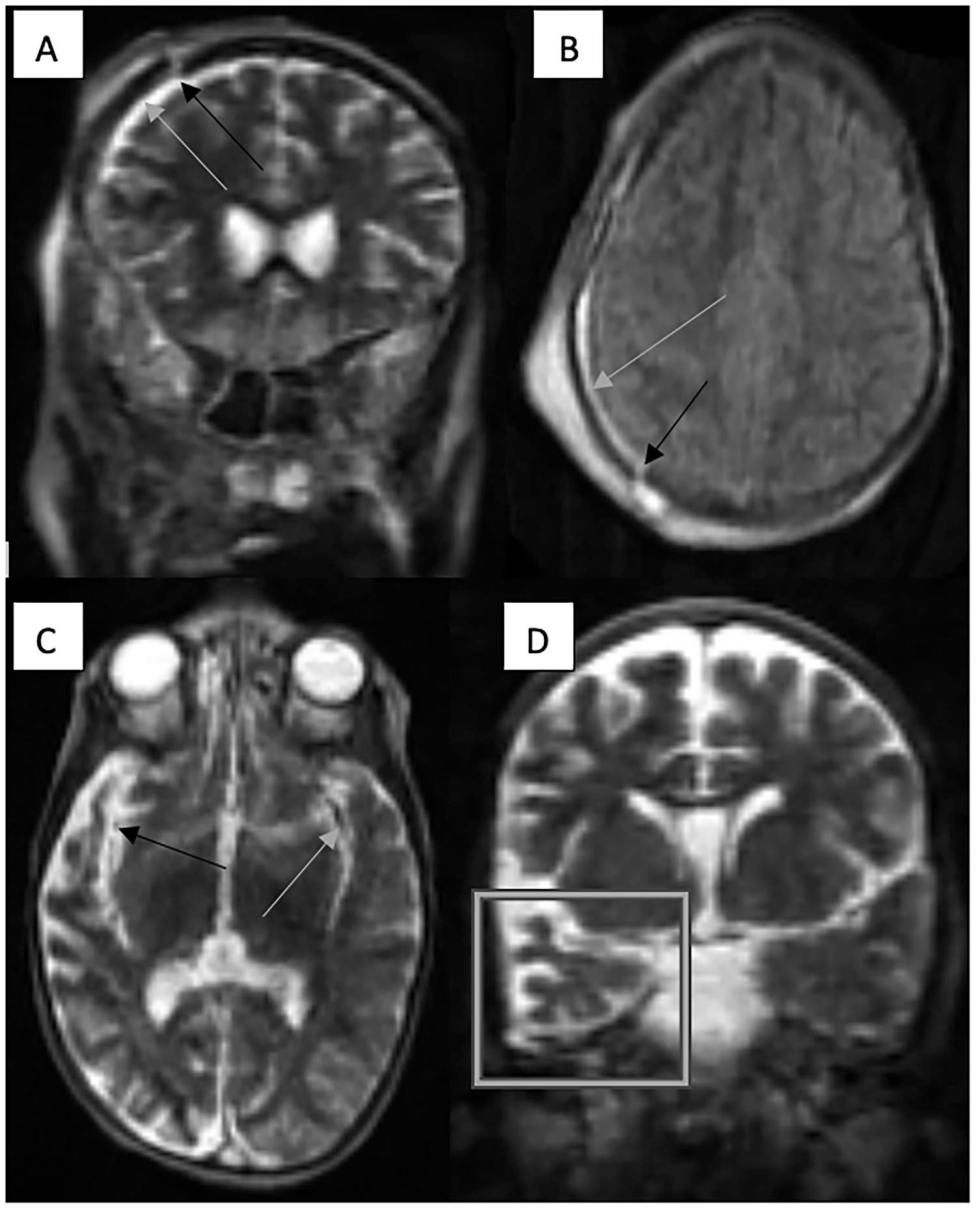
Post traumatic findings in Panels A and B and post ischaemic event sequelae in Panels C and D as imaged on the LF-MRI. Mildly depressed right frontal skull fracture (indicated by the black arrows in Panels A and B) with an overlying scalp oedema/haematoma and underlying widening of the underlying T2W and FLAIR hyperintense subdural fluid space (suspected to be a haematoma) (indicated by the grey arrows in Panels A and B) on the coronal T2W and axial FLAIR sequences. Global atrophy of the grey and white matter in the right temporal lobe (indicated within the grey box in Panel D) on a background of reduced brain volume for the patient’s age with suspected asymmetry with narrowing of the right middle cerebral artery (indicated by the black arrow in Panel C) as compared to its counterpart on the left (indicated by the grey arrow in Panel C) on the axial T2W sequence.
